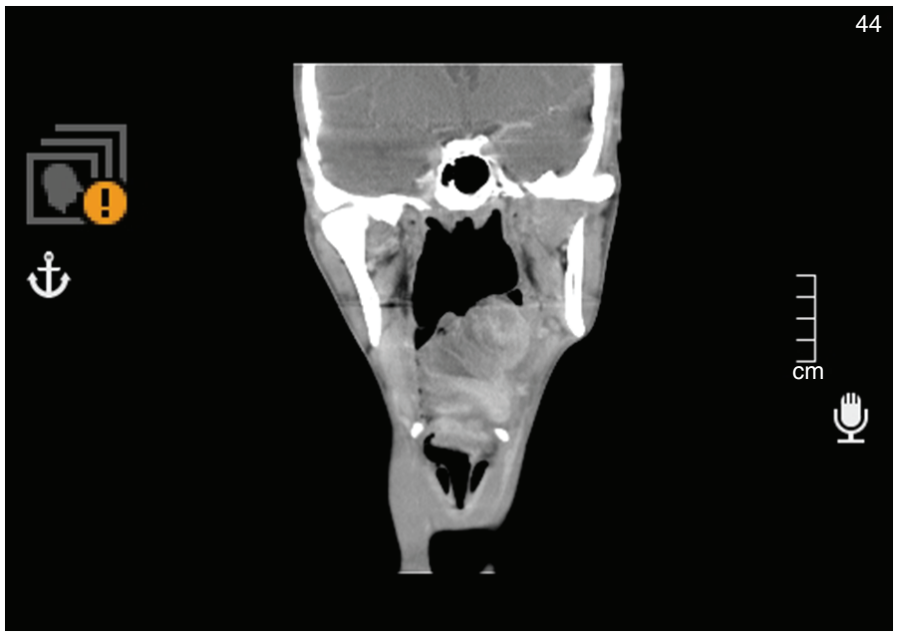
CT scan showing large left oropharyngeal tumor extending from the lower end of the left palatine tonsil to the junction with hypopharynx.
